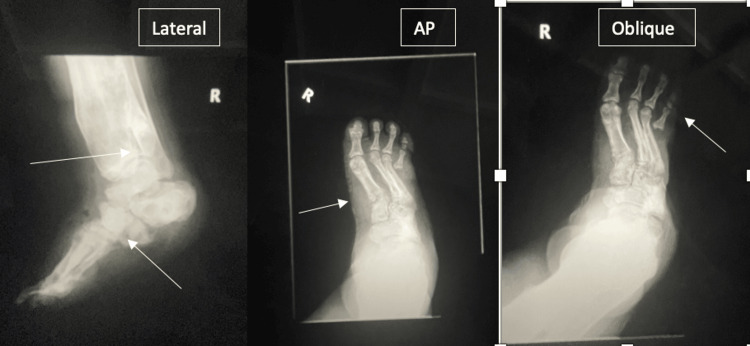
Lateral, AP, and oblique X-ray images of the patient’s right foot. In the lateral image, the top arrow indicates prior fractures of the distal tibia and fibula, while the bottom arrow highlights Charcot changes of the midfoot with gross deformity of the mid- and hindfoot. In the AP image, the arrow points to soft tissue swelling over the dorsal foot, concerning for soft tissue infection. In the oblique image, the arrow indicates bony absence of the fifth digit.
